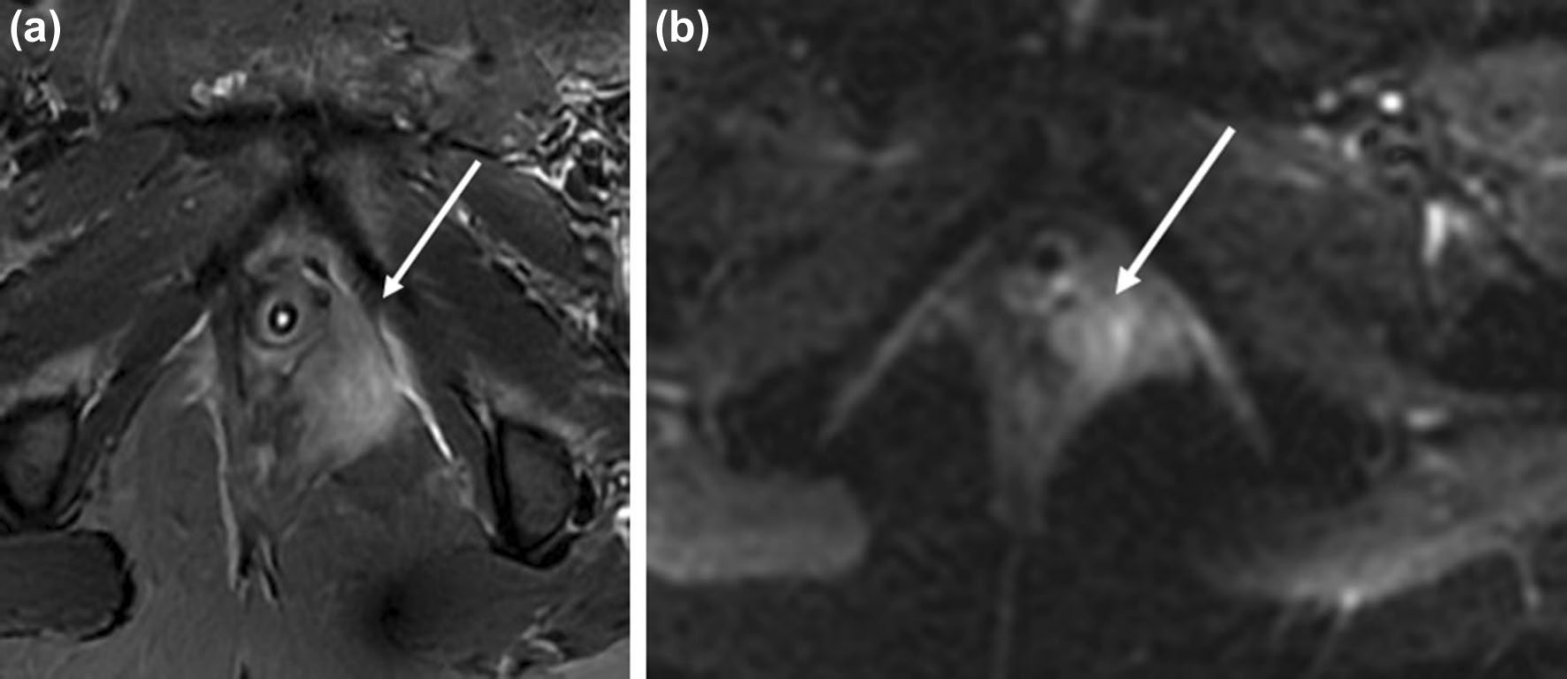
48-year-old woman with recurrent squamous cell carcinoma of the vagina. a Axial T2-weighted fat-saturated MR image shows a hyperintense mass within the left perineum causing mass effect on the left lateral vaginal wall (arrow) and extending into the left periurethral space. b DWI shows the lesion (arrow) with restricted diffusion
